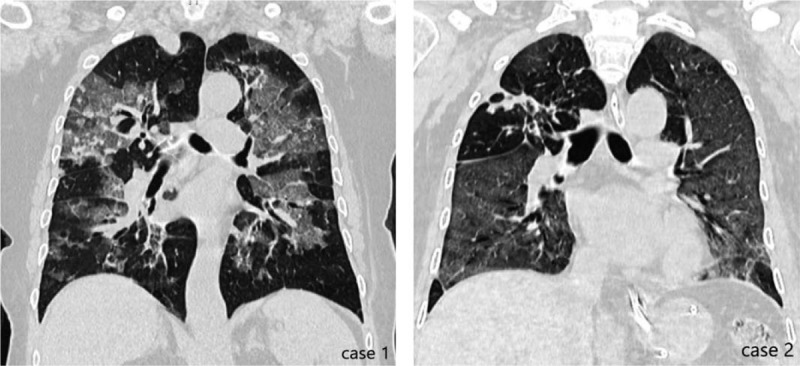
Chest CT scans of case 1 and case 2 when they entered ICU. CT = computed tomography, ICU = intensive care unit.
